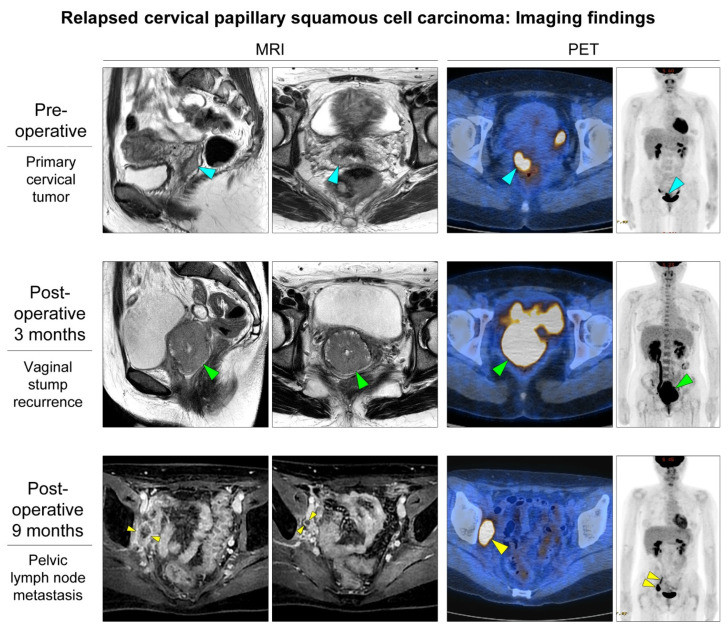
Imaging findings of a 33-year-old woman with a history of abnormal cervicovaginal cytology and high-risk human papillomavirus infection. Preoperative sagittal and axial magnetic resonance imaging (MRI) reveal an exophytic cervical mass (blue arrowheads) that seem to protrude into the vaginal lumen. The parametrium, lymph node, and adnexa are unremarkable. Positron emission tomography (PET) reveal a hypermetabolic lesion in the right lateral aspect of the uterine cervix (blue arrowhead), which is also shown in the maximum intensity projection image. She underwent radical hysterectomy with bilateral pelvic lymph node dissection and was diagnosed with early-stage cervical squamous cell carcinoma. Three months postoperatively, follow-up MRI and PET detect a recurrent tumor on the anterior wall of the vaginal stump (green arrowheads). She received chemoradiation therapy and achieved complete remission. However, pelvic lymph node metastases are developed nine months postoperatively (yellow arrowheads). Axial MRI and PET show two enlarged and hypermetabolic lymph nodes in the right obturator area.
